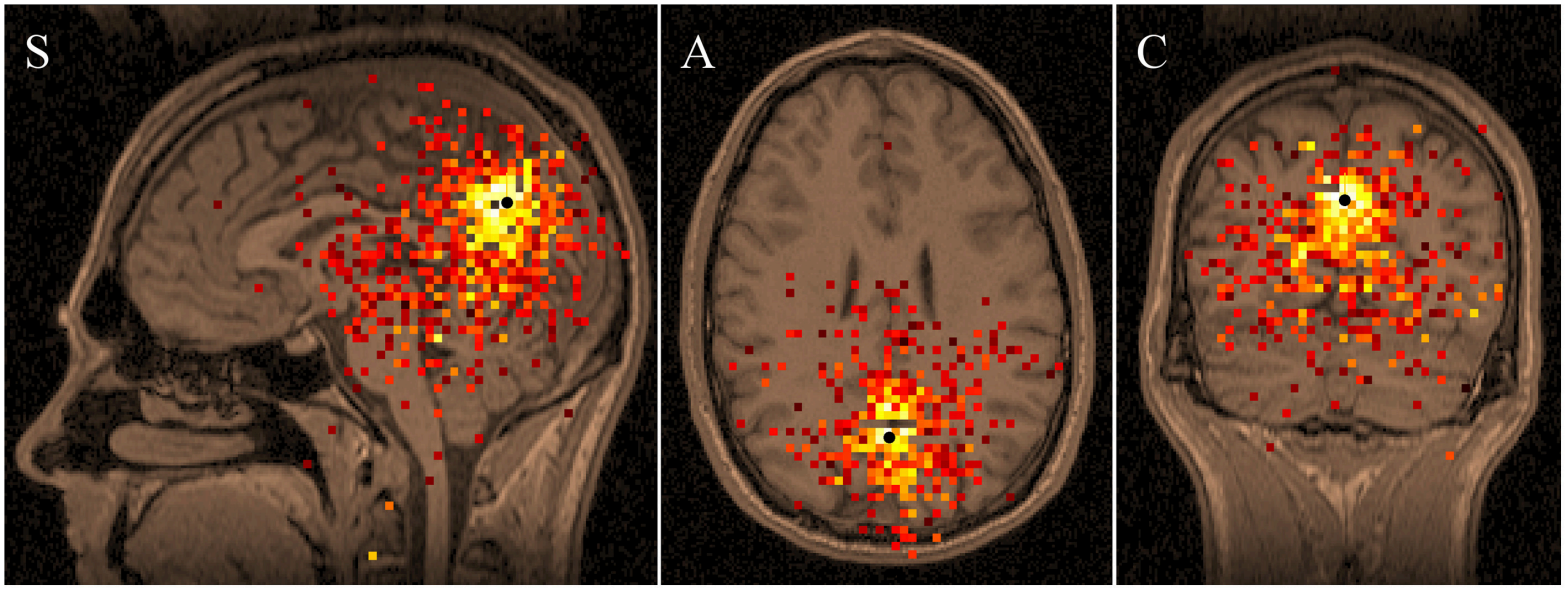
Average “Eyes Closed” functional tomogram of alpha band activity recorded from 10 individuals, plotted over the MRI from subject #5. Sagittal (S), axial (A), and coronal (C) tomographic sections are shown.
